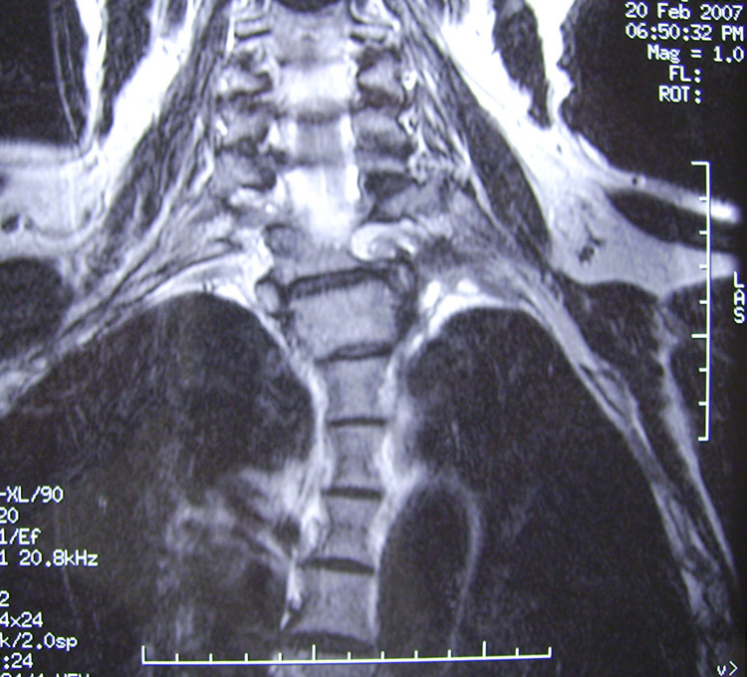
Coronal section of T1 weighted MRI demonstrating complete removal of the left-sided T1-neuroma from the T1-2 intervertebral foramen and from the pulmonary apex.
